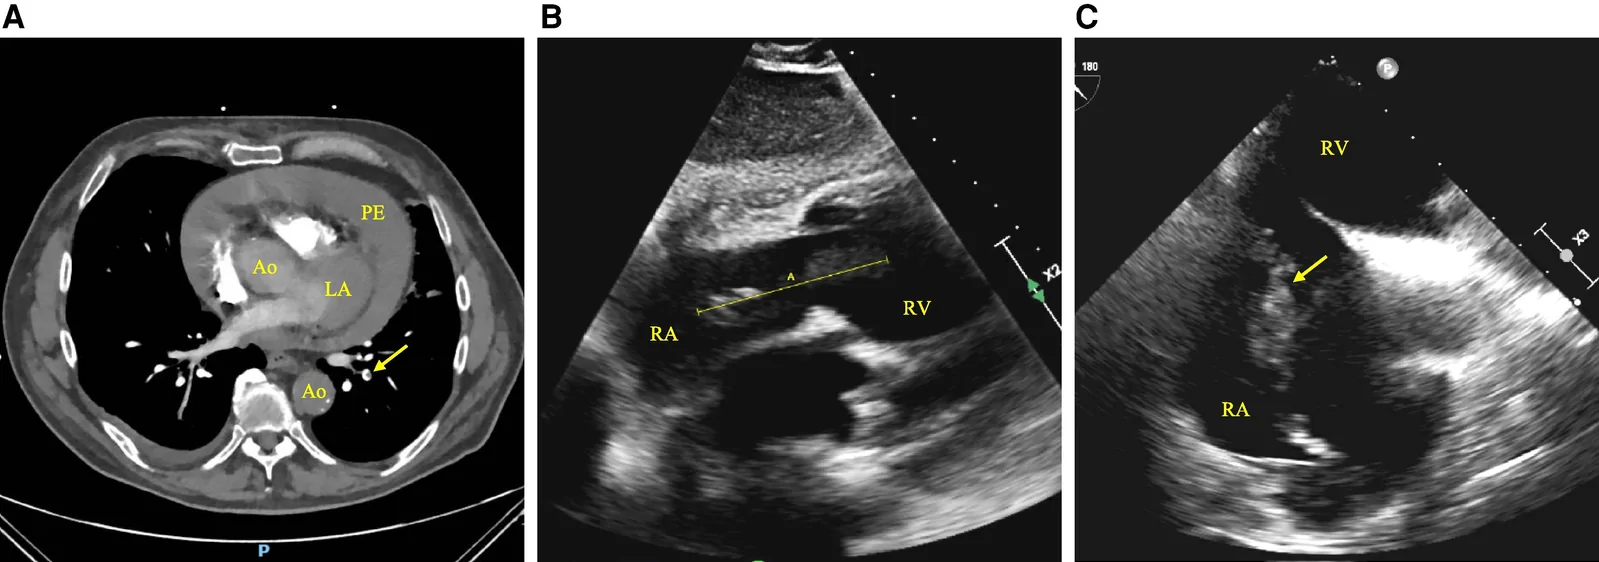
Imaging at first presentation at referring hospital. (*A*) Computed tomography demonstrating large pericardial effusion and segmental pulmonary embolisms (arrow). (*B*) Transthoracic echocardiogram showing a large mobile mass (6.5 cm) in the right atrium. (*C*) Transoesophageal echocardiogram showing a pedunculated mass originating from the right atrial appendage (arrow). Ao, aorta; LA, left atrium; PE, pericardial effusion; RA, right atrium; RV, right ventricle.
